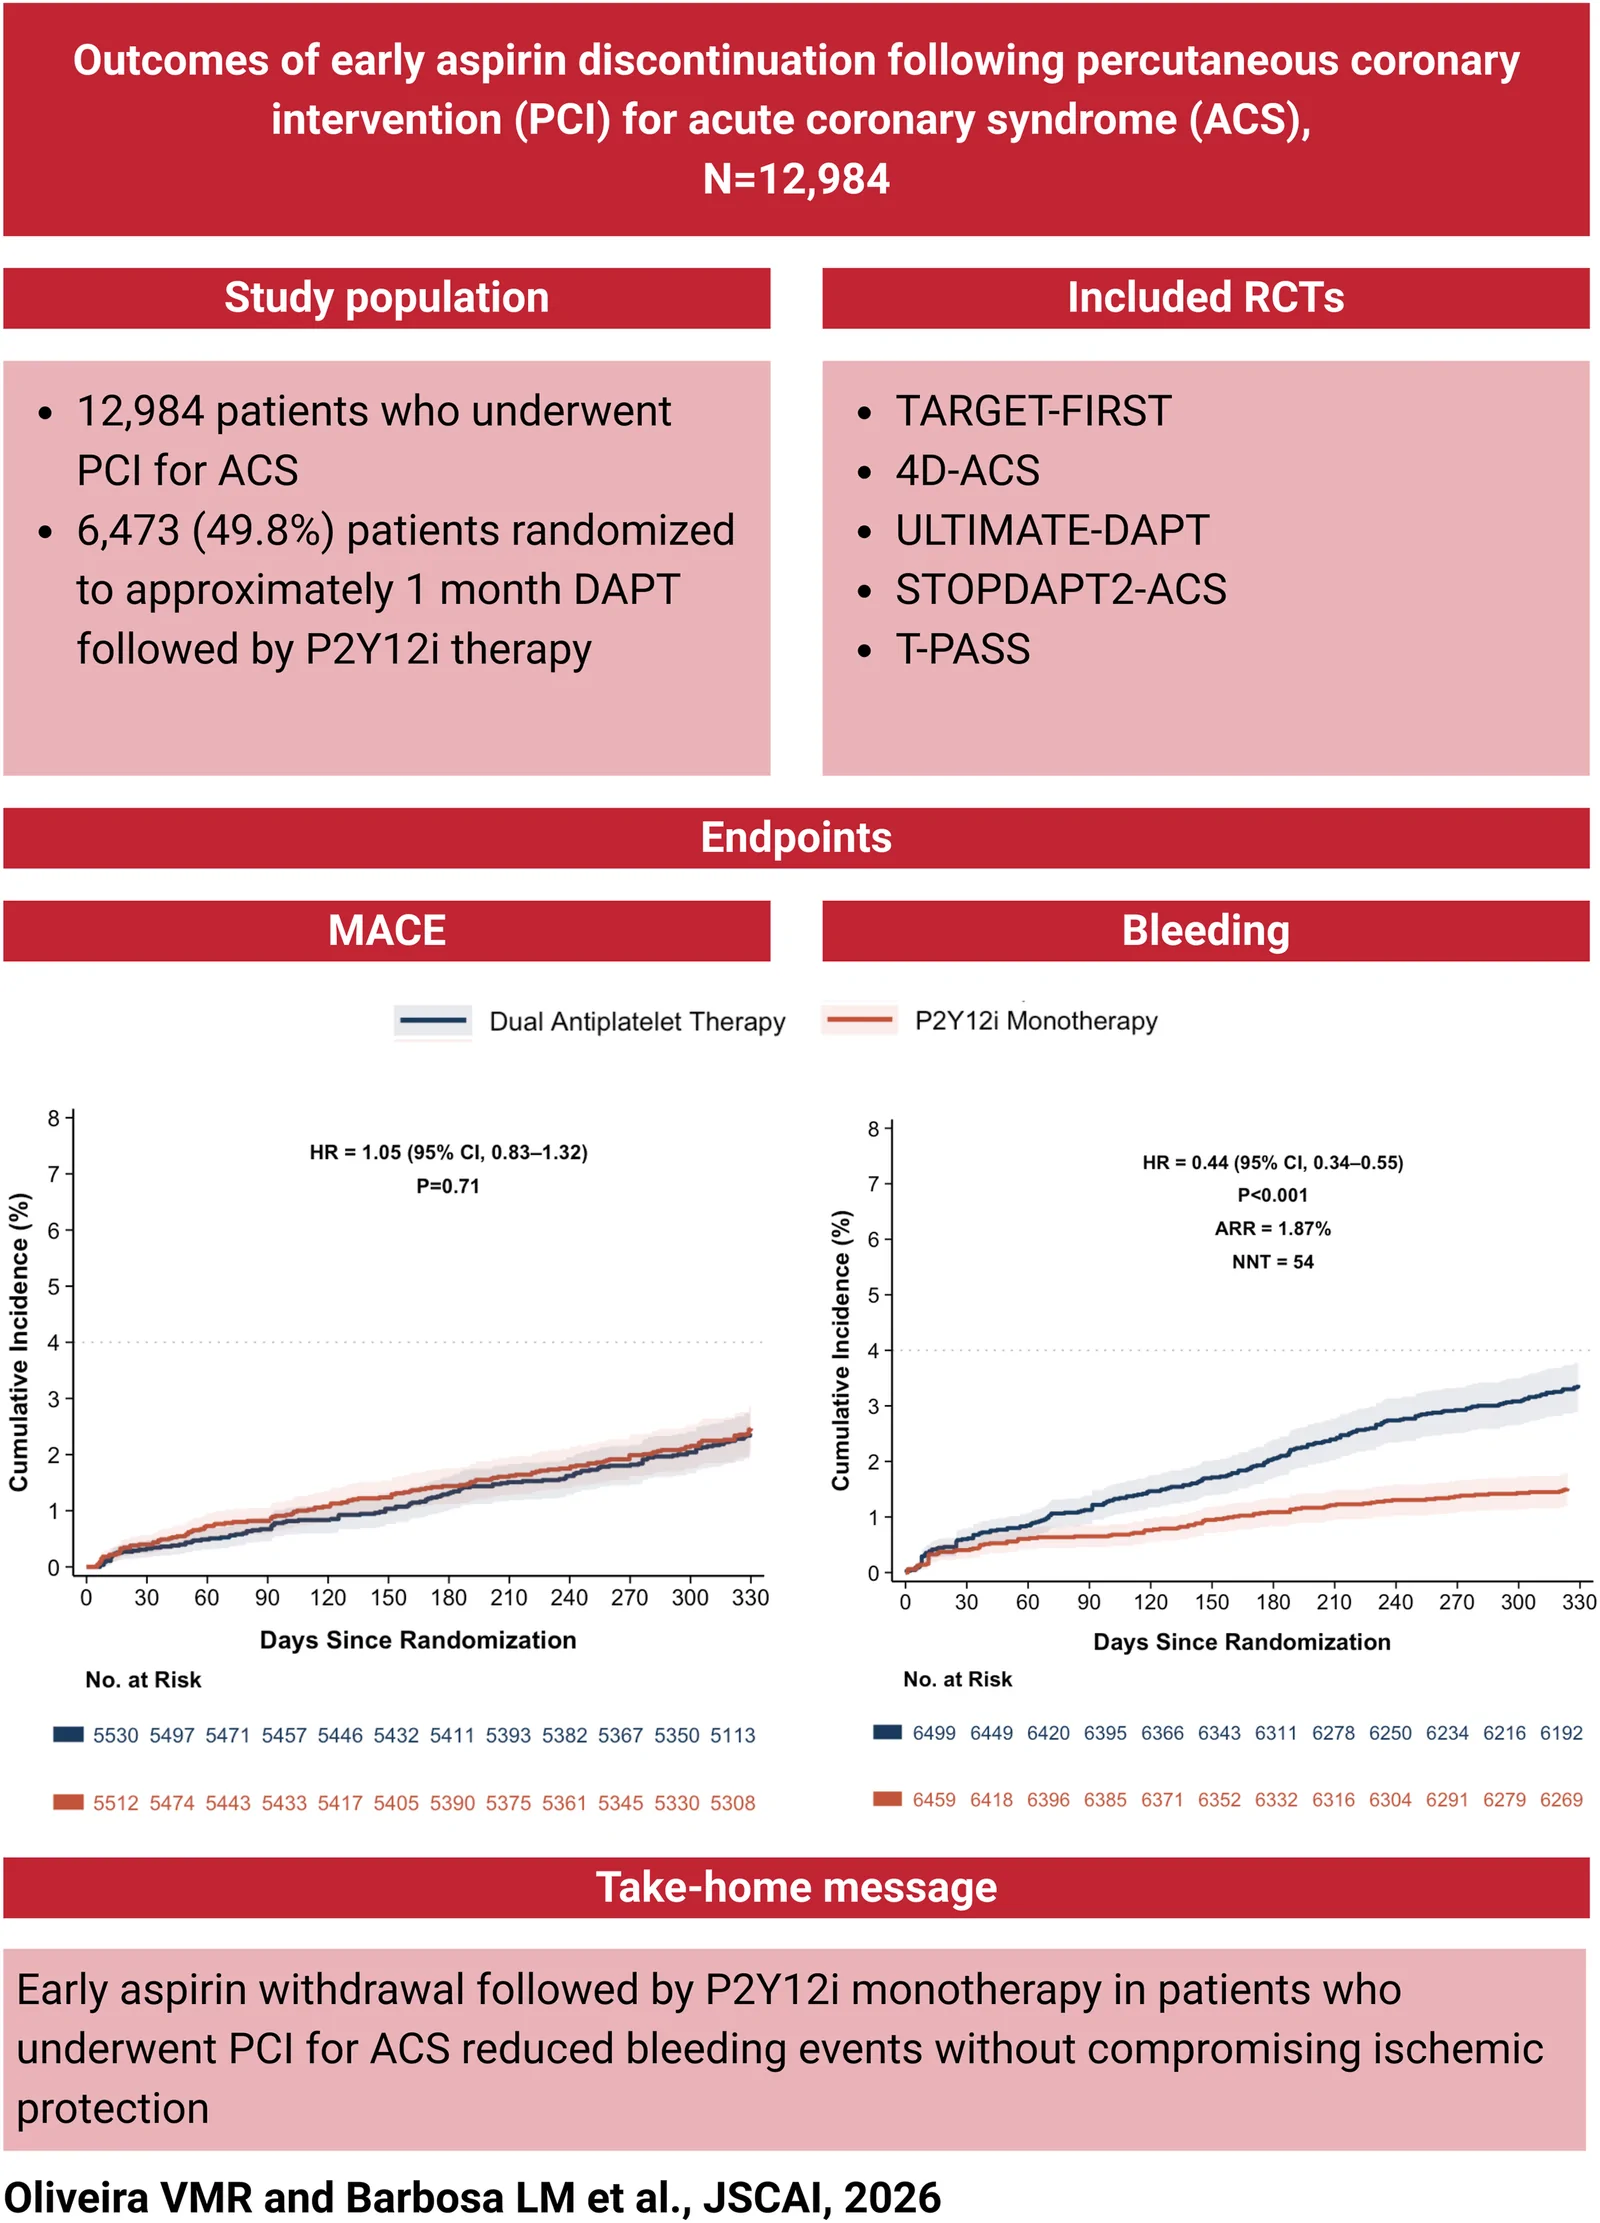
Earlyaspirin discontinuation followed by P2Y_12_i monotherapy after percutaneous coronary intervention (PCI) for acute coronary syndrome (ACS) significantly reduced bleeding events without compromising ischemic protection across 5 randomized controlled trials. ARR, absolute risk reduction; DCS, drug-coated stents; HR, hazard ratio; MACE, major adverse cardiovascular events; NNT, number needed to treat; STOPDAPT-2 ACS, Short and Optimal pciDuration of Dual Antiplatelet Therapy-2 Study for the Patients With Acute Coronary Syndrome; TARGET-FIRST, Evaluation of a Modified Antiplatelet Therapy Associated With Low-dose DES Firehawk in Acute Myocardial Infarction Patients Treated With Complete Revascularization Strategy; T-PASS, Ticagrelor Monotherapy in Patients Treated With New-Generation Drug-Eluting Stents for Acute Coronary Syndrome; ULTIMATE-DAPT, Comparison of 1 Month vs 12-month Dual Antiplatelet Therapy After Implantation of Drug-Eluting Stents Guided by Either Intravascular Ultrasound or Angiography in Patients With Acute Coronary Syndrome; 4D-ACS, The effect of short Duration of DAPT followed by Dose reduction after Implantation of DCS in ACS patients.
